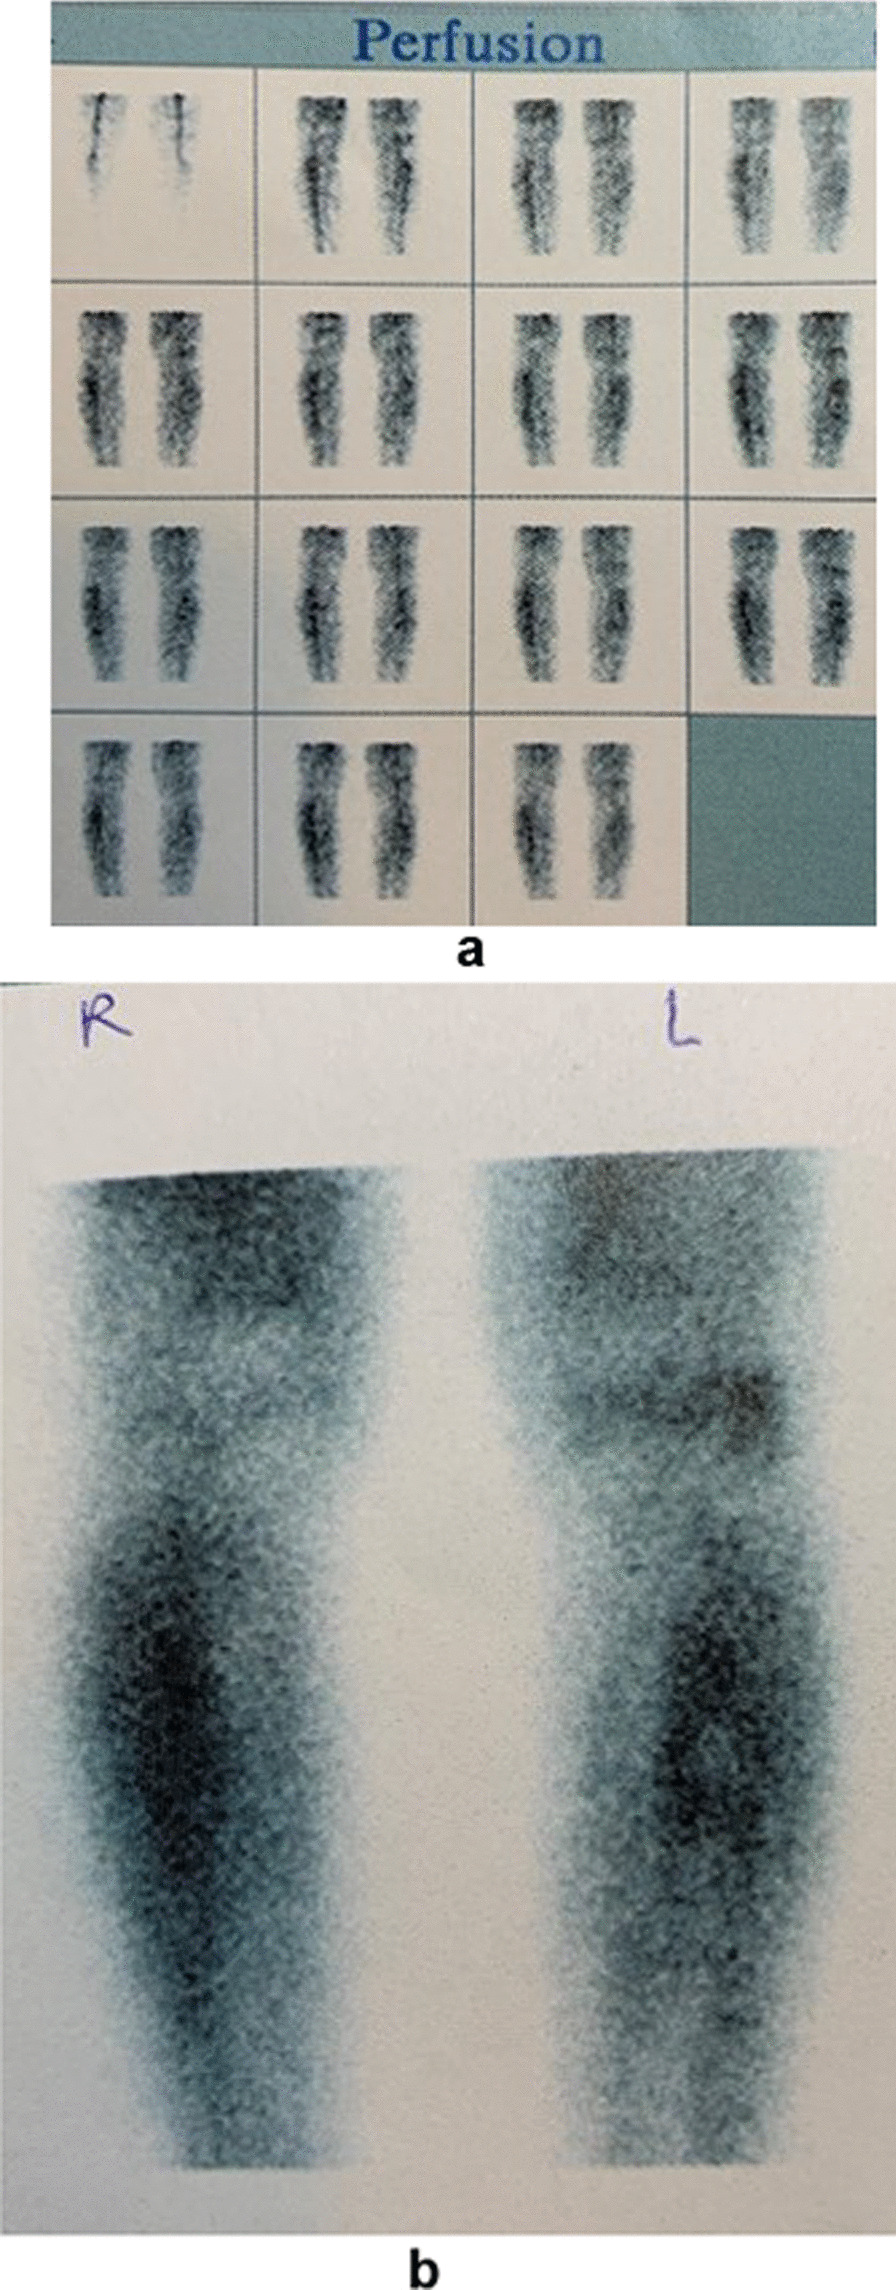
a, b Images depicting the bone scintigraphy of both patient’s tibiae with the same perfusion and signal
